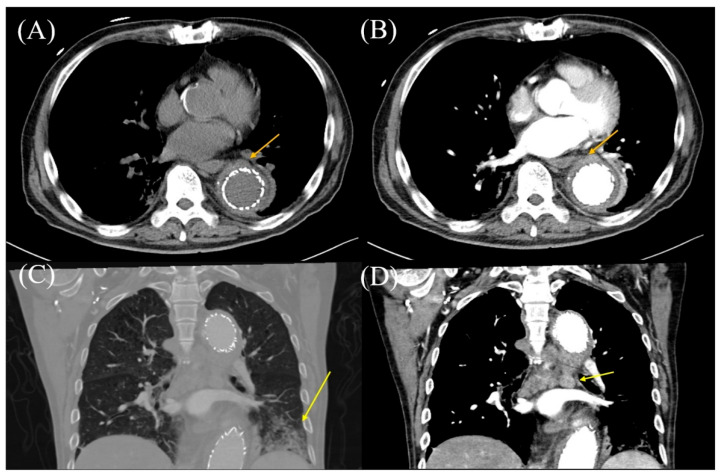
The results of computed tomography (CT) with or without contrast agent. (A) Thoracic CT without a contrast agent reveals that the aortic wall has Hounsfield units (HU) of 21 (arrow). (B) Thoracic CT with a contrast agent reveals areas of enhancement in the aortic wall, located outside the aortic stent, with an HU of 82 (arrow). (C) Thoracic CT in the coronal section reveals pneumonia (arrow). (D) Contrast-enhanced thoracic CT reveals mediastinal lymph node enlargement (arrow).
